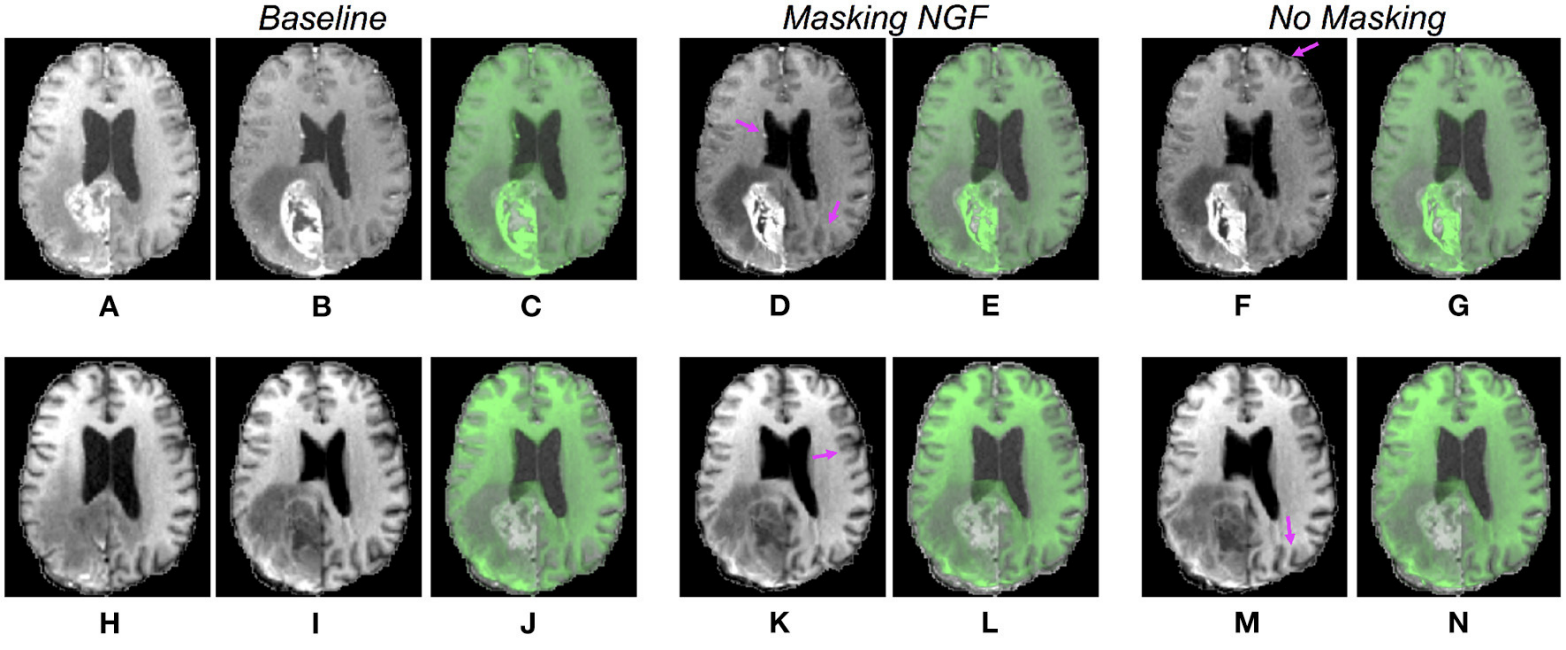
Comparison of qualitative results for CNN models trained on T1-CE and T1 MRI sequences. Each row refers to the results for the CNN models trained on different sequences (from the top to the bottom, T1-CE and T1). The post- and pre-operative images are visualized in the first and second columns (A,B,H,I), and the initial overlay between the two acquisitions is visible in the third column (C,J). The last four columns display the warped moving volumes (D,F,K,M) and the overlays between the fixed image (post-operative) and the warped moving images (E,G,L,N), respectively, for the models excluding and including the pathology in the distance measure. The purple arrows point to locations where improvements are observable. In panels (D,K), a better overlap of the lateral ventricles is visible. Moreover, in panels (F,K,D) the sulci are better registered.
